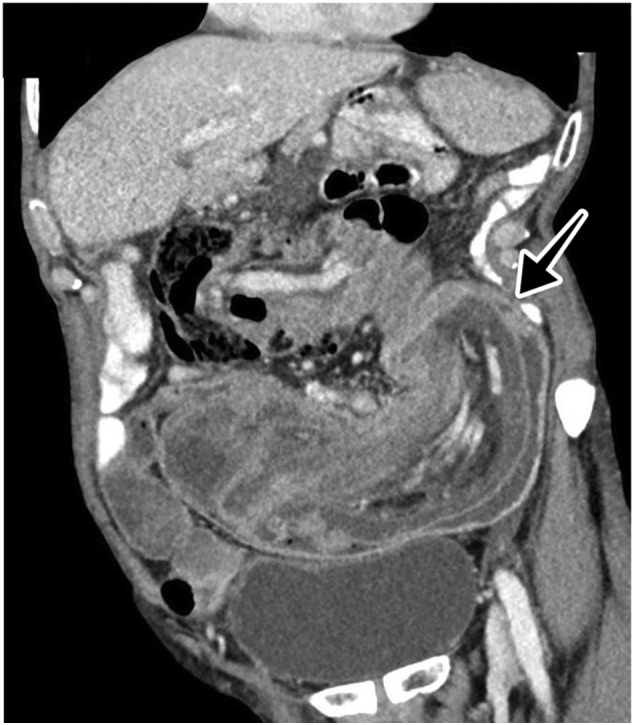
CT scan of the abdomen. Coronal plane showing an ileo-colic intussusception with classic ‘sausage-shaped mass’ (arrow).
